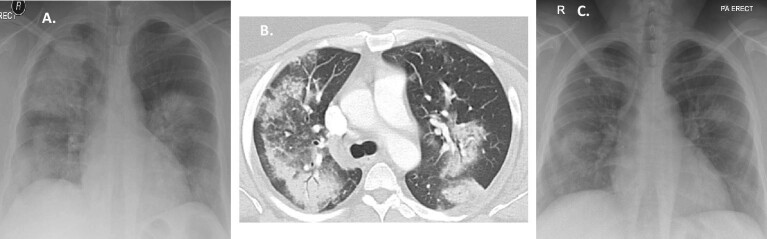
(A) Patient 8 at presentation: chest X-ray: bilateral consolidation; (B) Patient 8 at presentation: chest CT scan: diffuse ground-glass opacity of the lungs and areas of consolidation; (C) Patient 8 after 10 days: chest X-ray: significant improvement.
